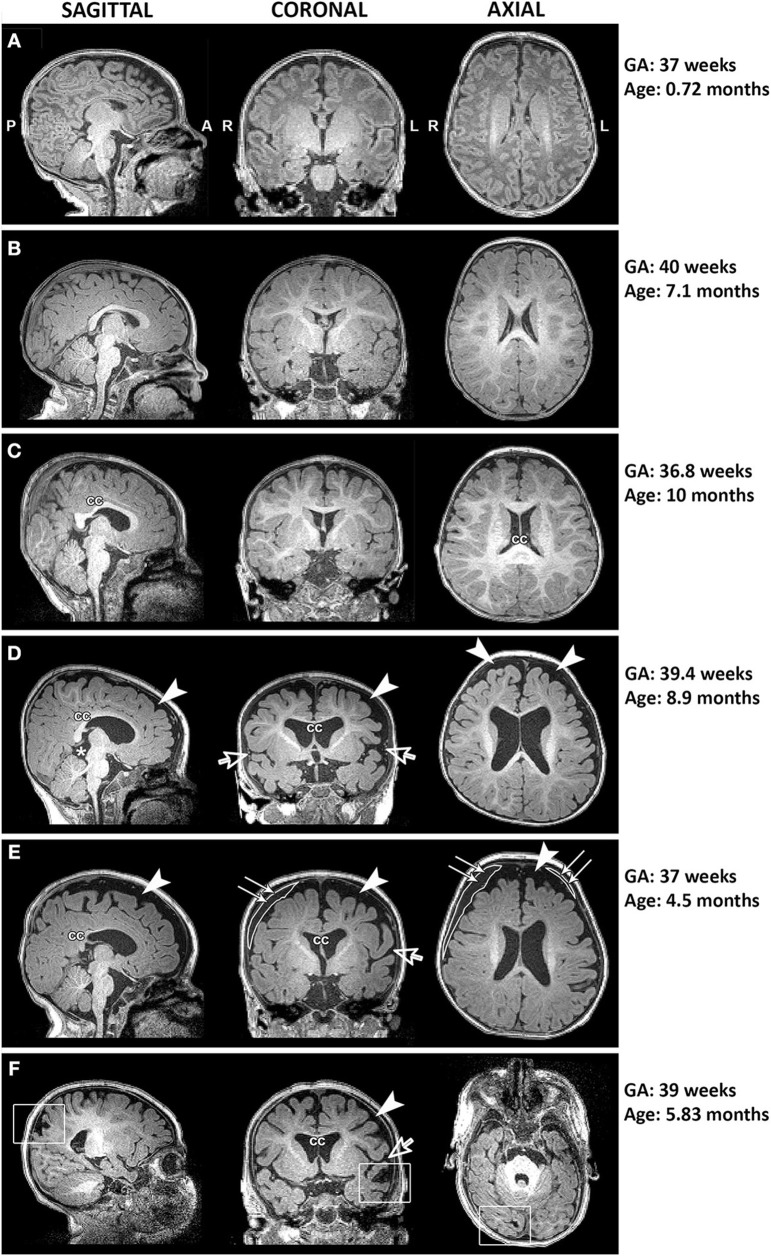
Representative T1-weighted images of full-term infants with typical brain structure (A,B) and those with altered corpus callosum (cc; C–F), increased extra-axial space (arrowheads) and widened Sylvian fissure (open arrows; D–F), presence of cyst (asterisk; D), incidental subdural hematoma (double arrows; E), and old venous hemorrhagic stroke (square boxes; F). L, left; GA, gestational age; R, right.
